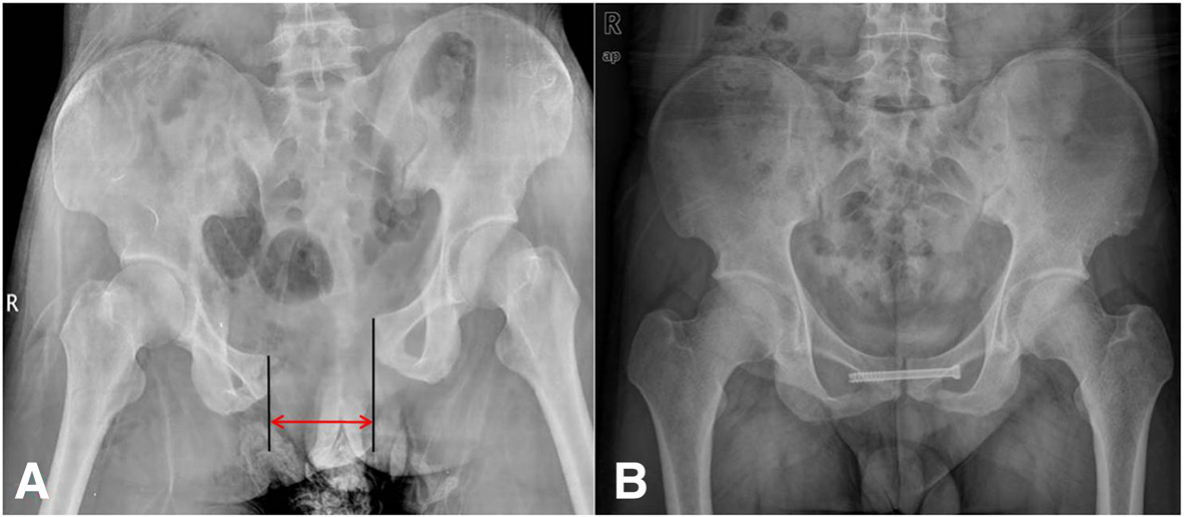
The radiographic images showed that one Tile B1 PSD patient (a) was treated with closed reduction and percutaneous cannulated screw fixation (b)
